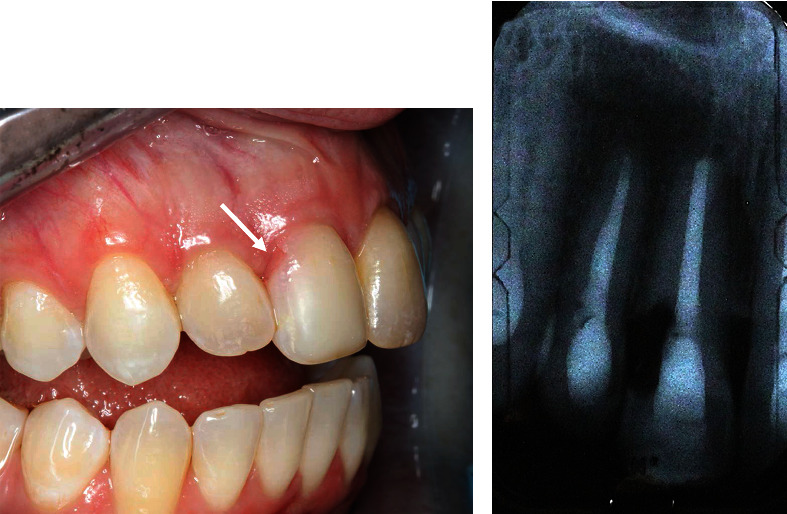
(a) Upper right central incisor with external root resorption (white arrow) was confirmed by (b) a periapical radiograph.
